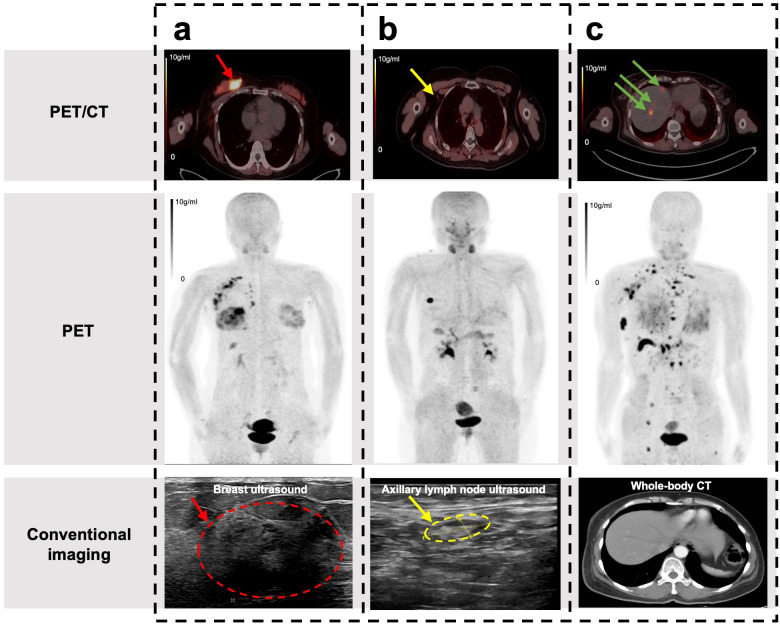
Typical [^18^F]AlF-NOTA-FAPI-04 PET/CT images used to detect breast cancers, regional lymph node metastases, and distant metastases. (a) Breast cancer was positive on FAPI PET/CT but classified as BI-RADS category 4a on conventional imaging. (b) Lymph node metastasis was detected on FAPI PET/CT but missed on conventional imaging. (c) Liver metastases were positive on FAPI PET/CT but not detected on conventional imaging.
